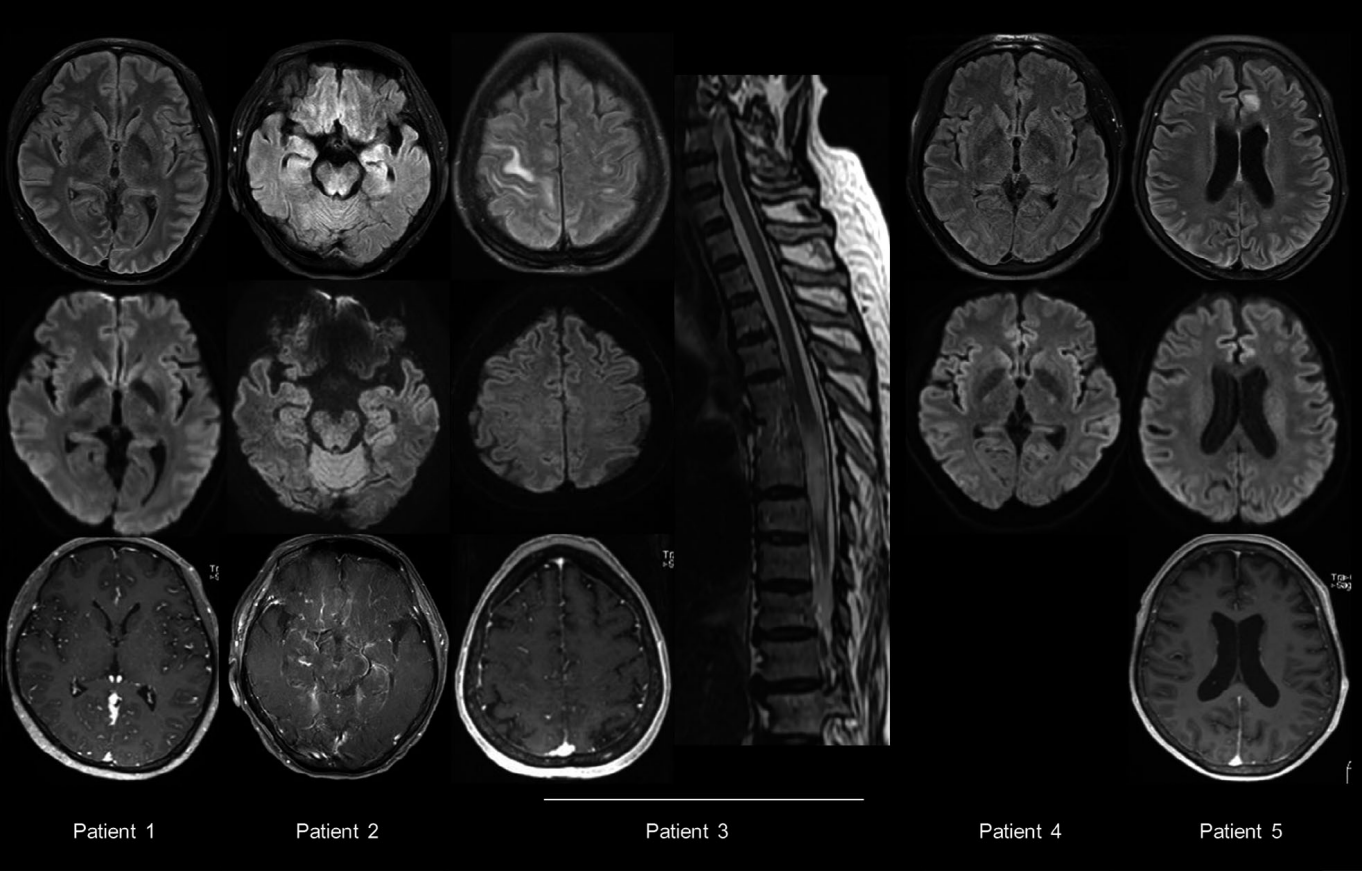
Lesions on MRI. Upper row: Diffusion‐weighted images. Middle row: Fluid‐attenuated Inversion Recovery Sequence. Lower row: Gadolinium‐enhanced T1‐weighted images, spine MRI of Patient 3: Ill‐defined T2 hyperintense intramedullary abnormality of the spinal cord at the T6 ~ T9 level.
